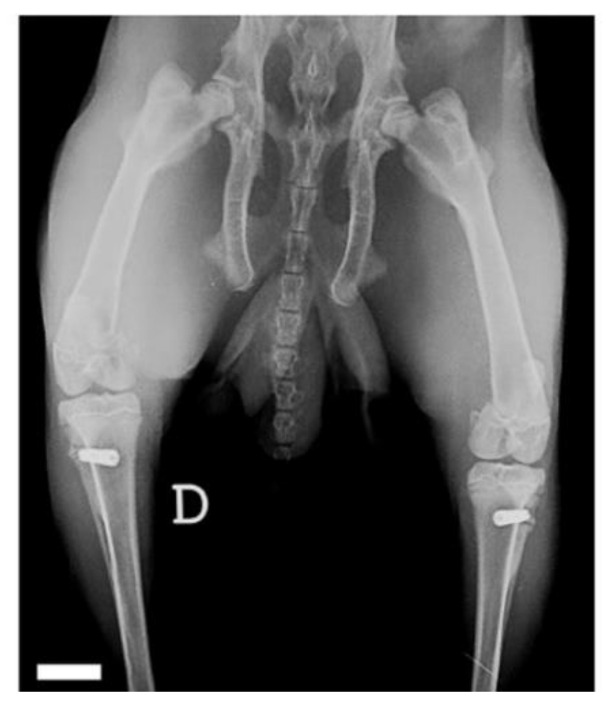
Final X-ray after implant placement surgery. Scale bar is 10 mm.
